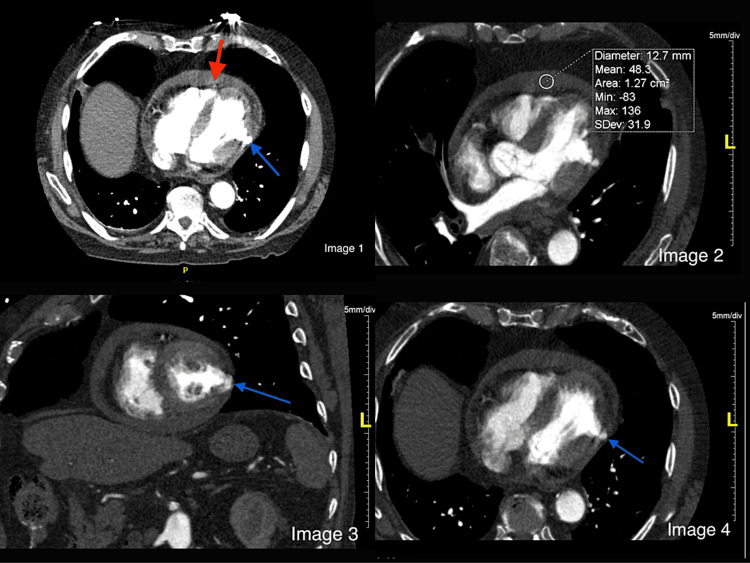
Image 1: CT chest axial section with the red arrow pointing to pericardial effusion and the blue arrow pointing toward a left ventricular aneurysm and potential rupture site. Image 2: CT chest three-chamber view with circle area of interest (AOI) revealing a mean fluid density of 48.3 Hounsfield units. Image 3: CT chest short-axis view of the heart with the blue arrow pointing toward a left ventricular aneurysm rupture site. Image 4: CT chest with the blue arrow pointing to left ventricular aneurysm rupture.
